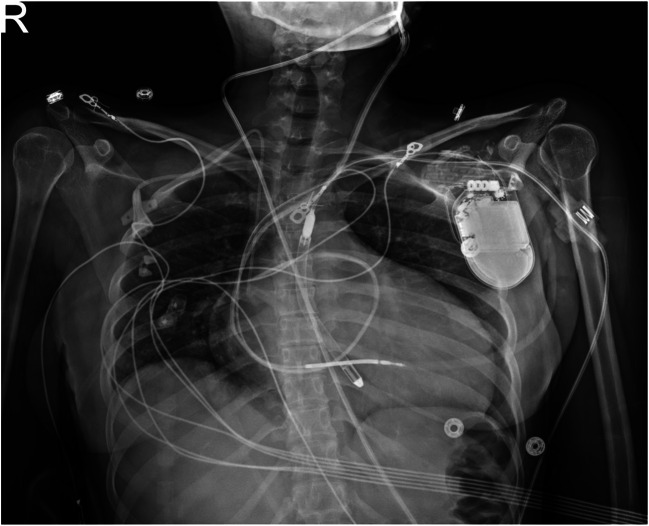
Chest x-ray with final placement of left axillary Impella 5.5
